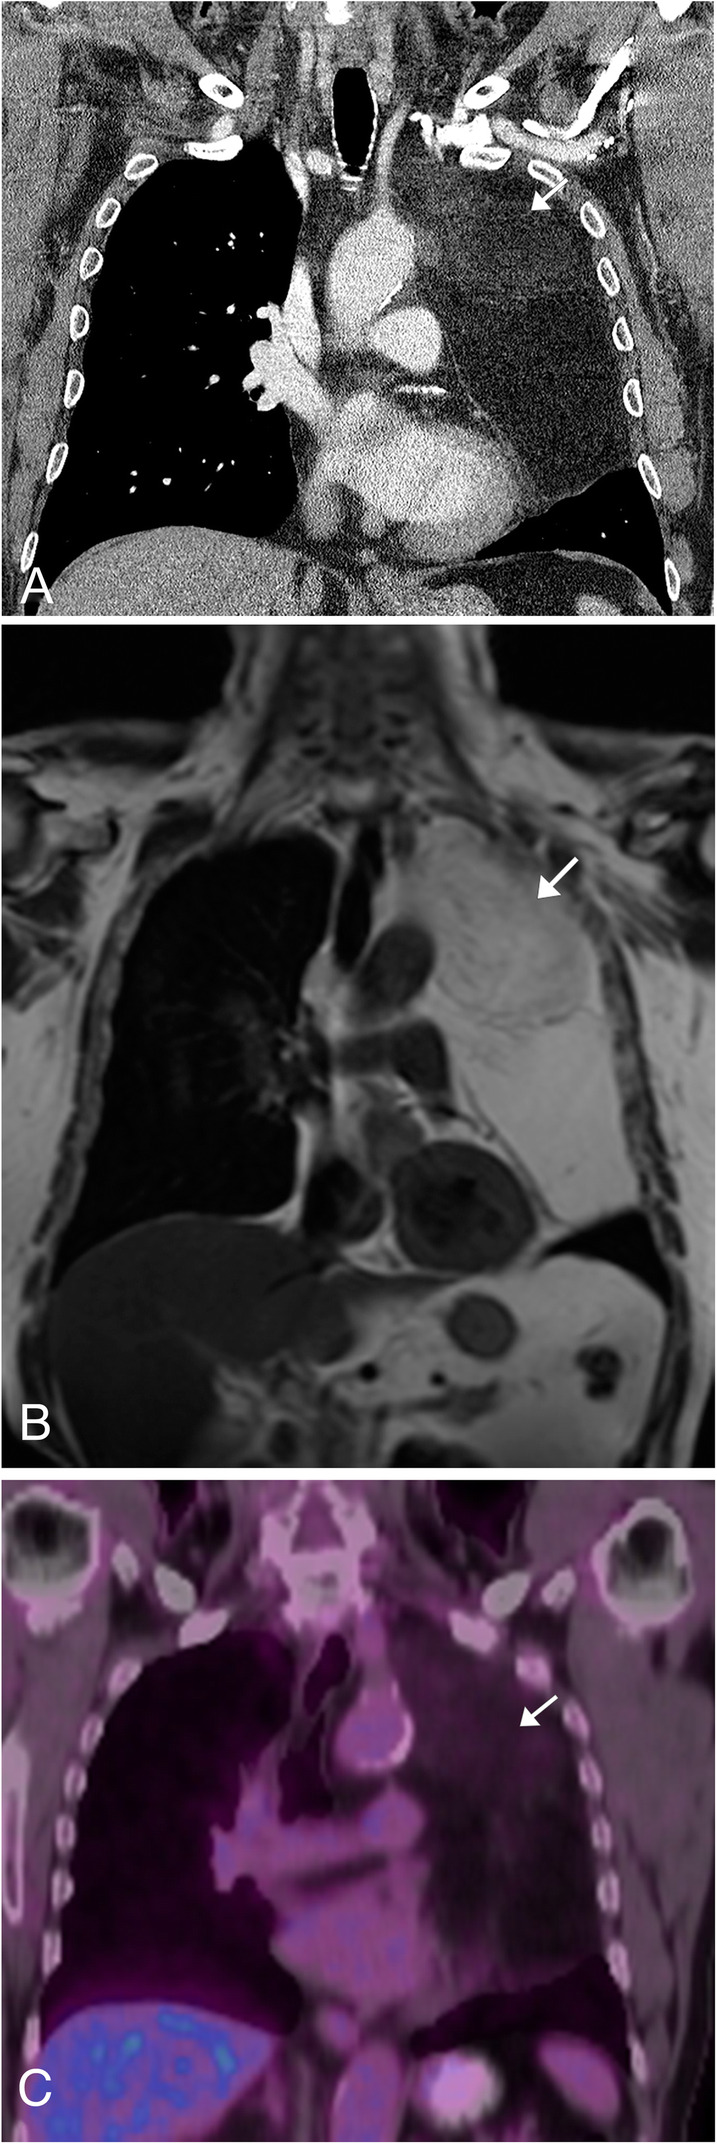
Liposarcoma. a Coronal CT image post contrast demonstrates a large low attenuation mass (fatty) located in the left upper hemithorax. The mass contains a heterogeneous area increased density/soft tissue component in the superior aspect of the mass (arrow), a finding which is suspicious for a liposarcoma. b Coronal T1WI MR images of the same lesion demonstrating a hyperintense mass in the left hemithorax with areas of heterogeneous low signal in the upper aspect of the mass (arrow). c Coronal FDG-PET/CT of the same lesion showing mild increased hypermetabolism in the area of heterogeneous enhancement within the fatty mass (arrow)
